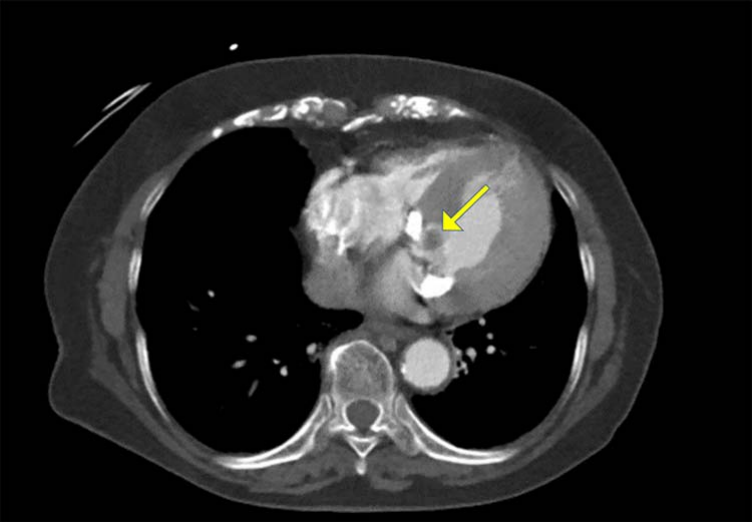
Cardiac computed tomography showing a cardiac mass in the mitral annulus with heavy mitral annular calcification.
